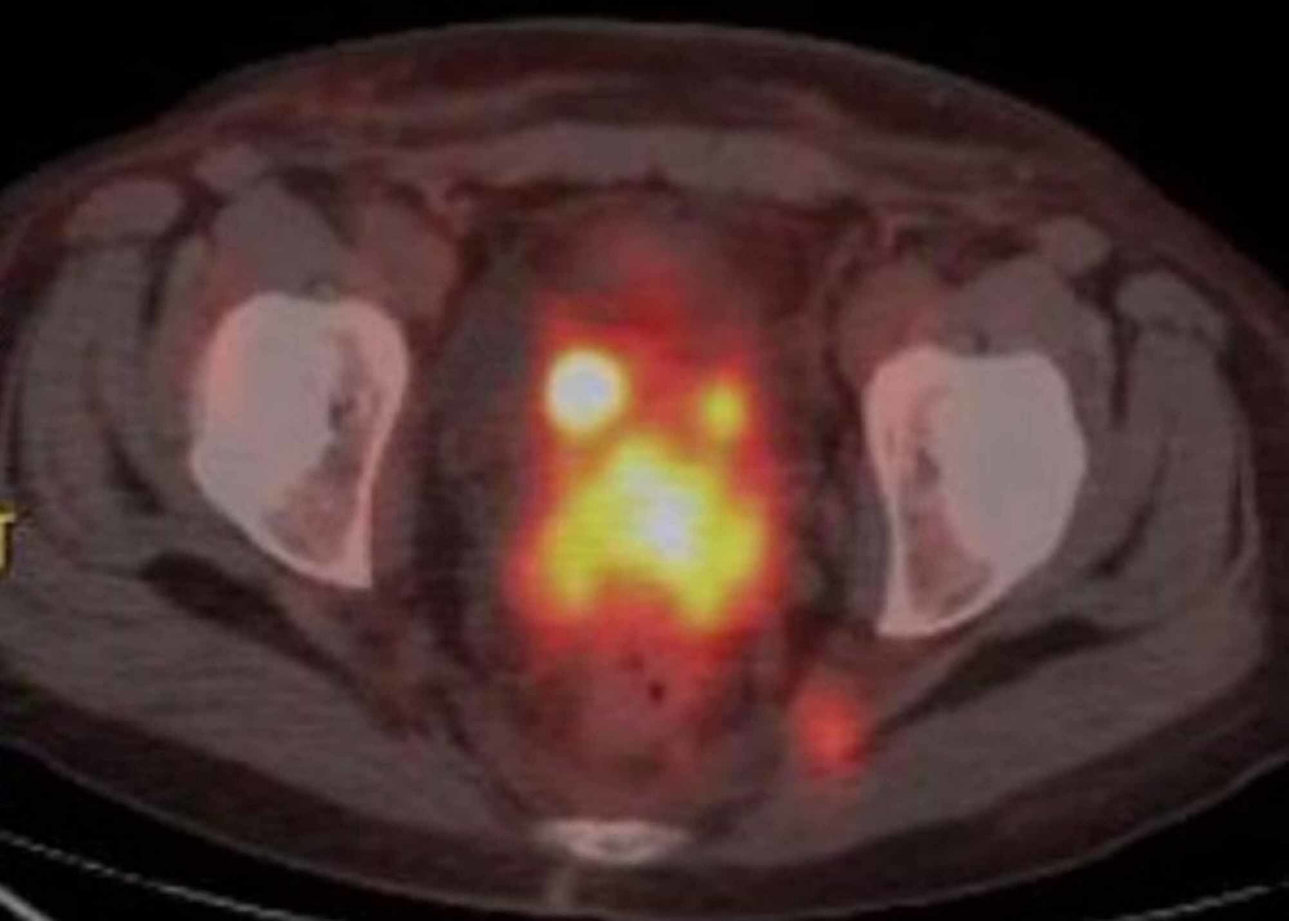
PET-CT scan of the pelvic shows focal area of marked hypermetabolic activity in the cervix PET-CT: positron emission tomography-computed tomography
